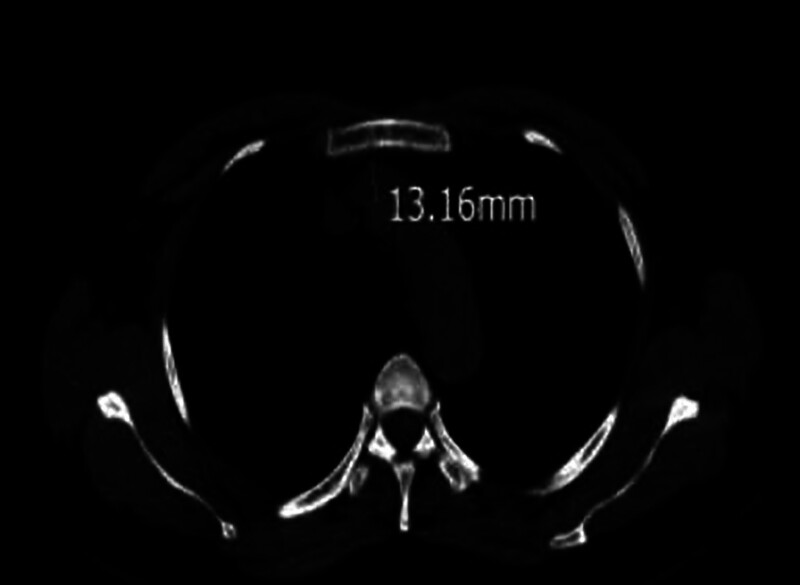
A 53-year-old woman with right-side breast cancer. An axial computed tomography (CT) image before totally implantable venous access port (TIVP) placement shows no evidence of left innominate vein stenosis.
